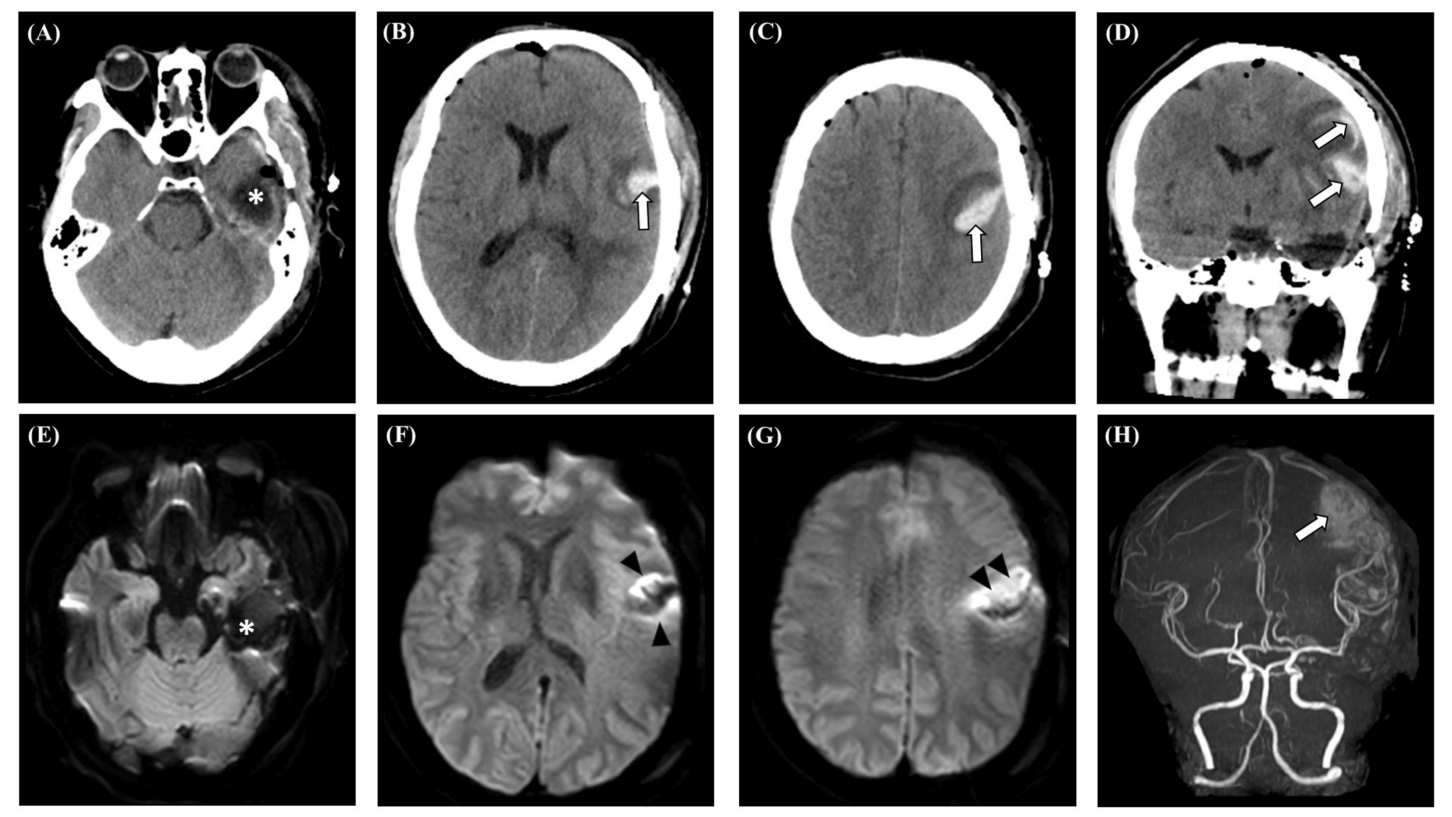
Postoperative imaging findings Postoperative emergent CT of axial (A–C) and coronal (D) images revealed intracerebral hemorrhage around the left precentral gyrus and inferior frontal gyrus (arrows), accompanied by surrounding low-density areas. The asterisks indicate the surgical cavity. Brain MRI performed 12 hours after surgery demonstrated heterogeneous diffusion-restricted areas (arrowheads) at a site distant from the extraction site (asterisks) on diffusion-weighted imaging (E–G), indicating remote hemorrhagic infarction. MRA of the frontal view revealed the presence of major arteries (H). CT: computed tomography; MRA: magnetic resonance angiography; MRI: magnetic resonance imaging
